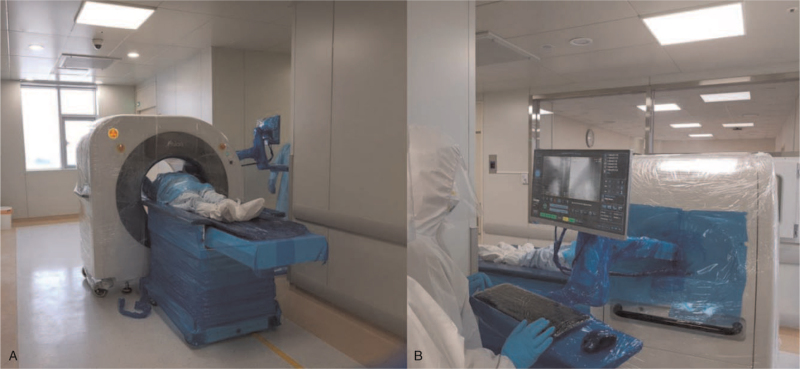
Mobile CT set up in the hallway in front of the isolation room. (A) Scene of COVID-19 patient mobile CT scan. (B) Scene of radiologic staff manipulates the mobile CT. COVID-19 = coronavirus 2019, CT = computed tomography.
